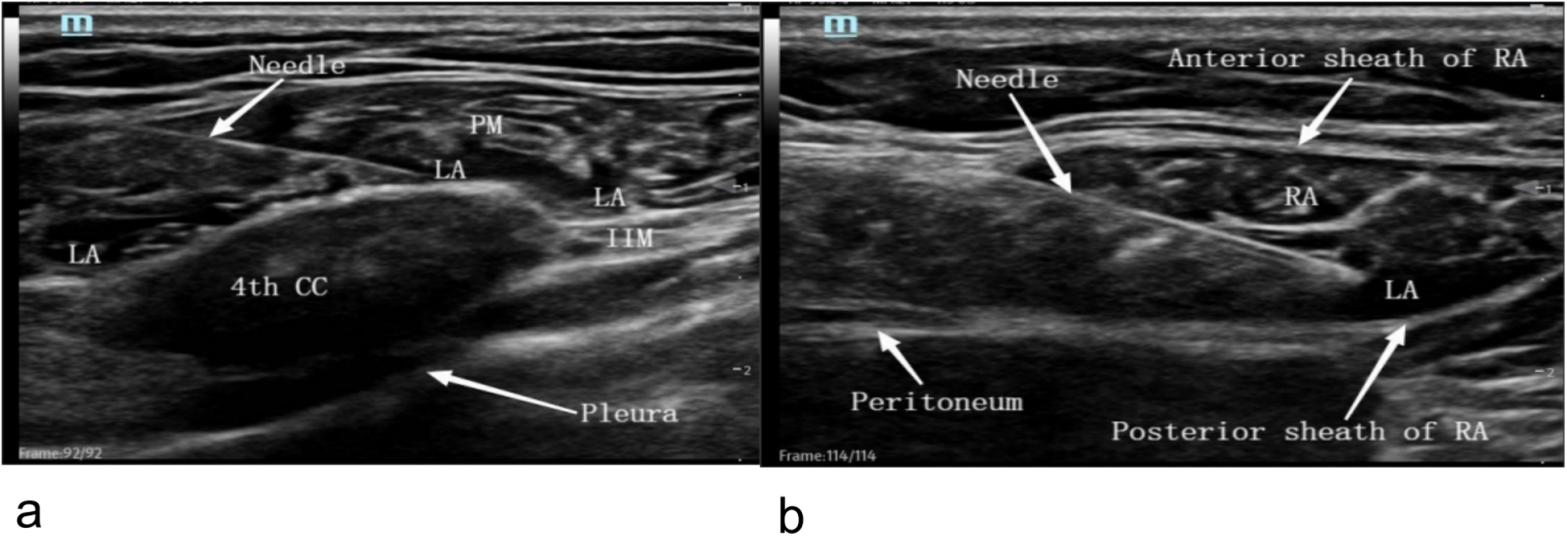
Ultrasound-guided block image. (a) parasternal block, (b) rectus abdominis sheath block. 4th CC, fourth costal cartilage; IIM, intercostal muscle; LA, local anesthetic, Needle, block needle; PM, pectoralis major; Pleura, pleura. Anterior sheath of RA, anterior sheath of rectus abdominis; LA, local anesthetic; Needle, blocking needle; Peritoneum, peritoneum; Posterior sheath of RA, posterior sheath of rectus abdominis; RA, rectus abdominis.
